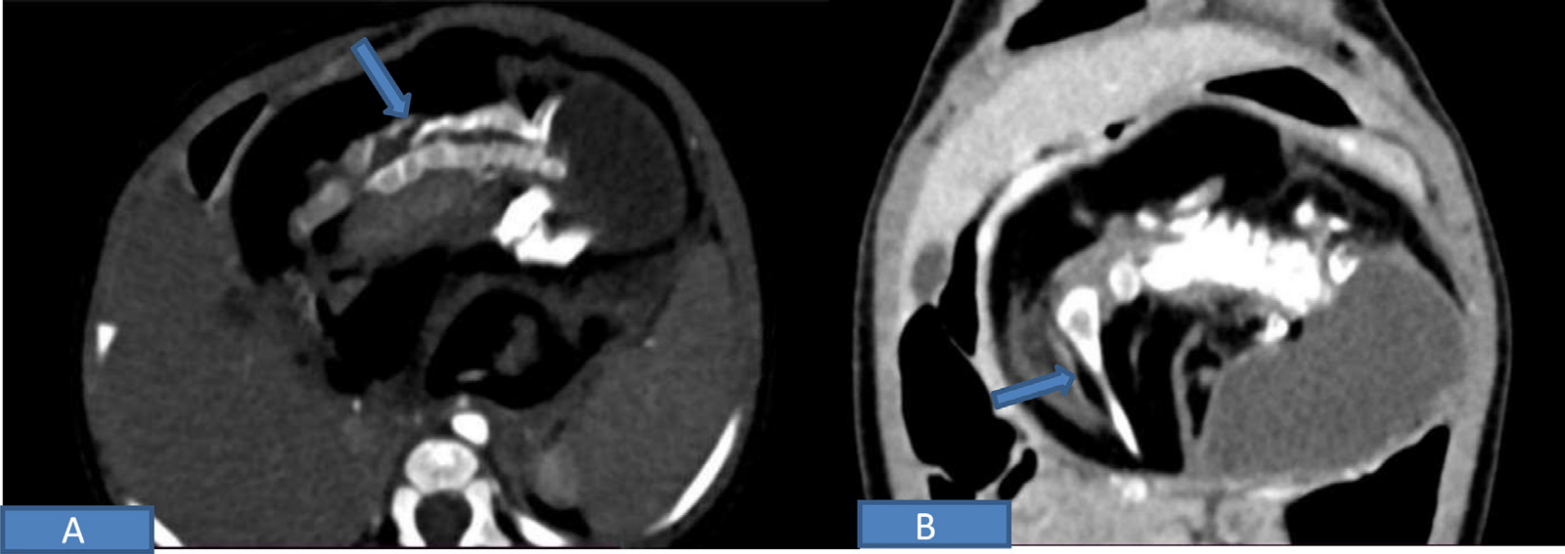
Contrast enhanced CT image* demonstrates heterogeneous mass containing fat and calcified content. (A) axial section shows fat attenuation in lesion with well differentiated bones of vertebral column and (B) shows coronal reconstruction with the arrow's points to the corticated limb bone.
